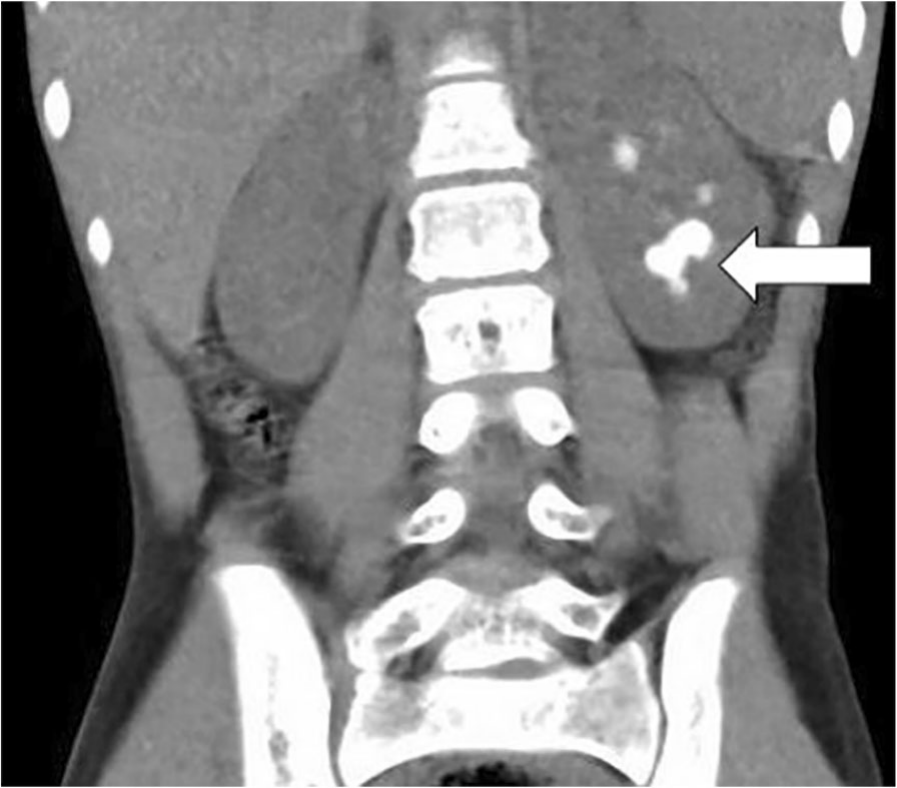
CT scan of the abdomen and pelvis showed staghorn calculi in the left kidney
